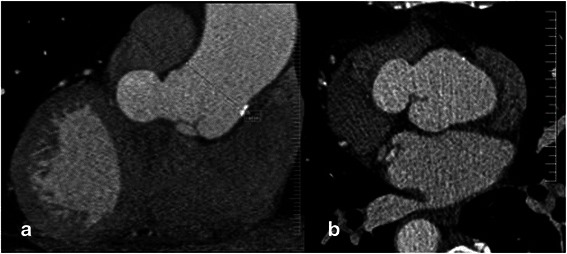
CT scan showing the right coronary sinus aneurysm in the sagittal (a) and axial plane (b)
